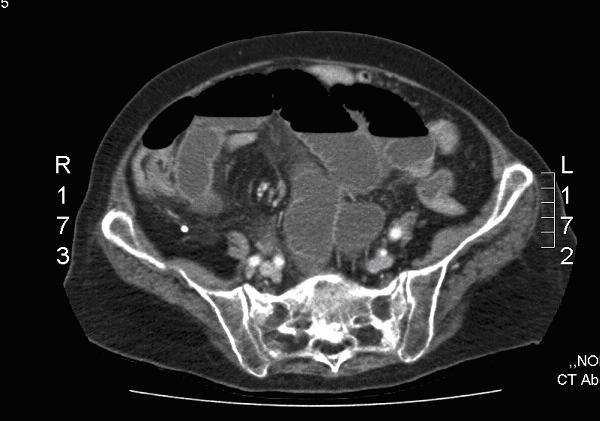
Abdominal CT scan revealing small bowel obstruction together with mural thickening of the distal ileum.
